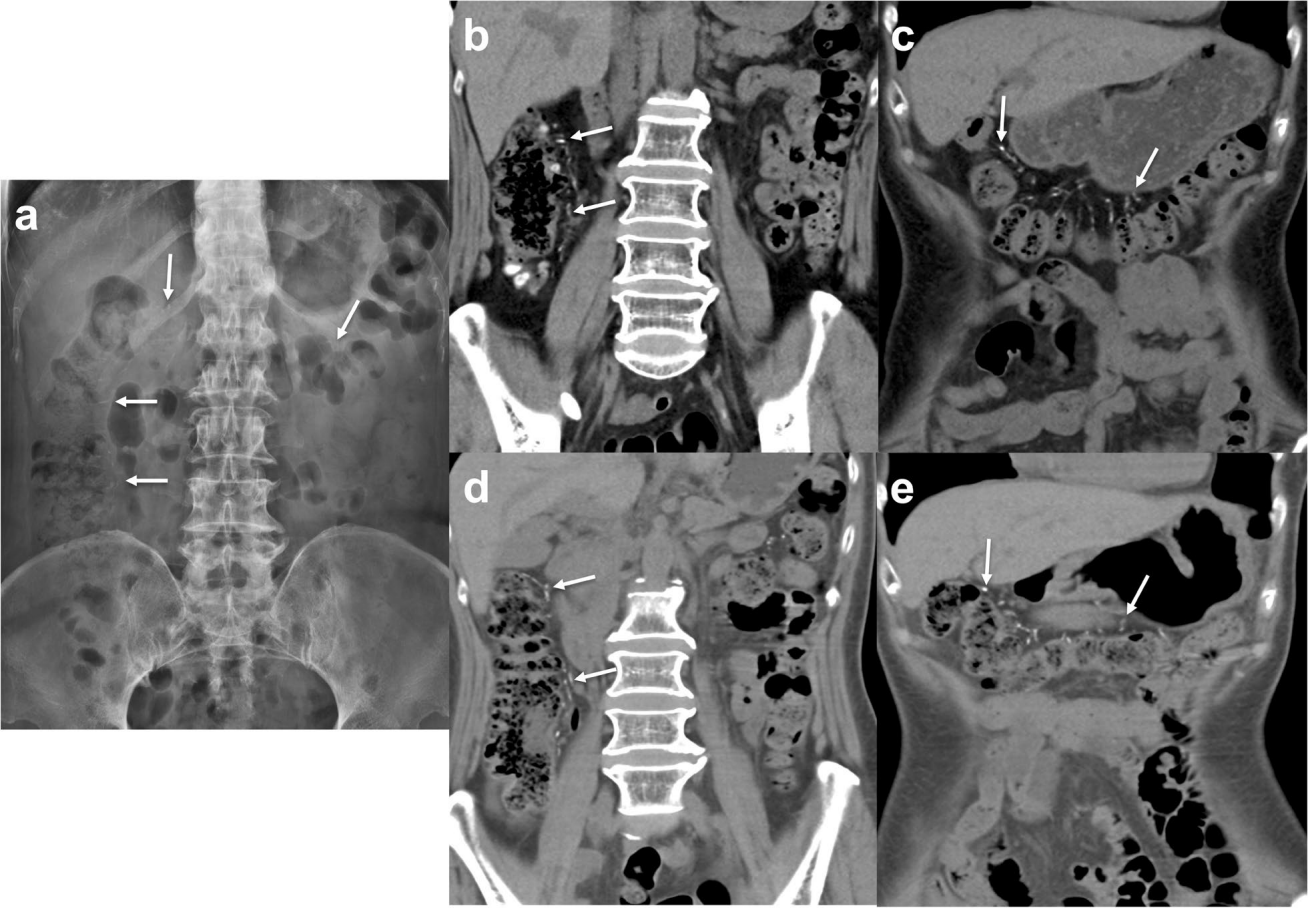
A 52-year-old asymptomatic woman had a past history of hysterectomy for early endometrial cancer. a Curvilinear calcifications alongside the right colon were incidentally observed on abdominal radiograph. b, c CT confirming calcified straight and marginal veins of the right colon without colitis. d, e Five-year follow-up CT shows stable calcified mesenteric veins with no colonic wall thickening
